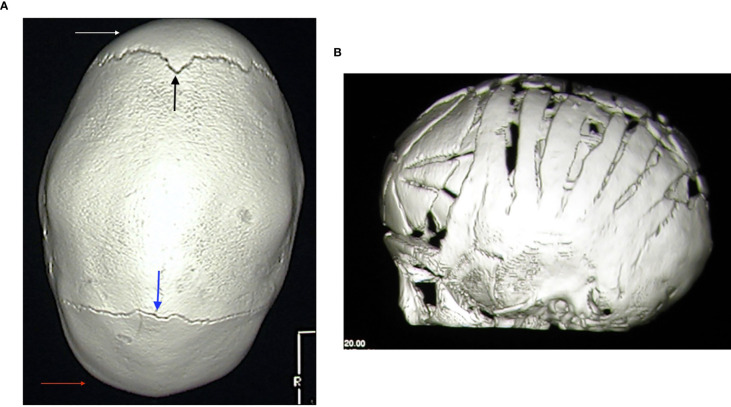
(A) 3D-reformatted axial computed tomography scan showing fusion of the sagittal suture and classic scaphocephalic head shape in a 3.2-year-old male patient with XLH. Frontal (white arrow) and occipital (red arrow) bossing with open coronal (black arrow) and lambdoid (blue arrow) sutures is also evident. (B) 3D-reformatted axial computed tomography scan showing the neurosurgical correction of scaphocephaly in a 8-month-old male patient with XLH.
